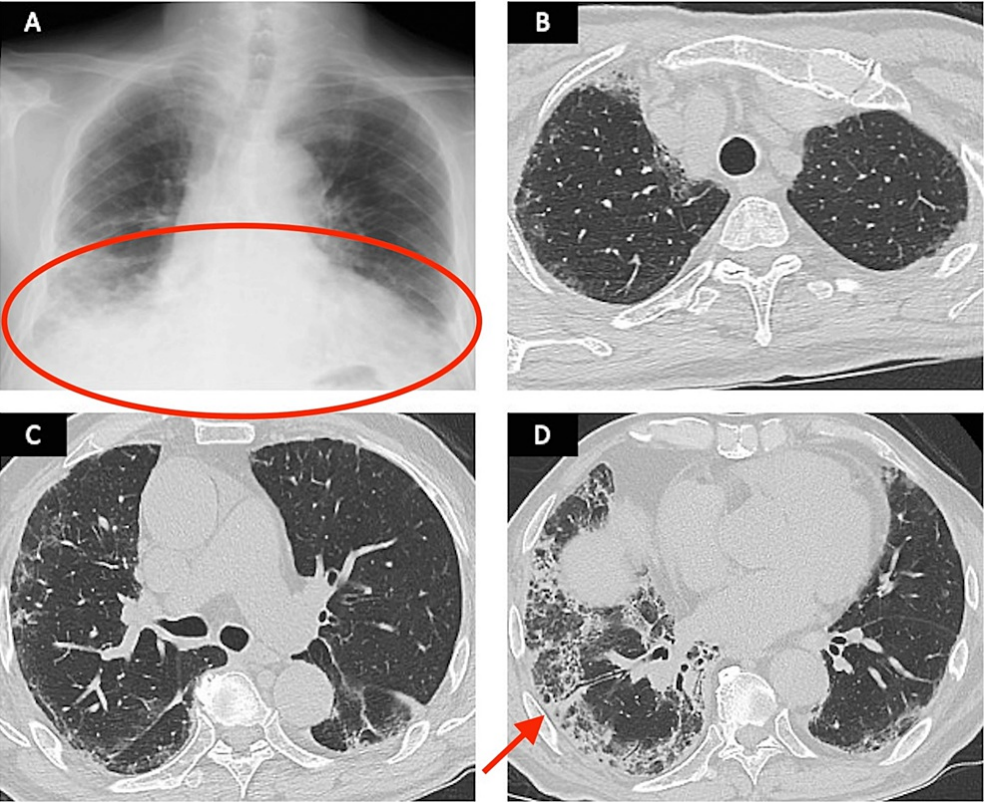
Initial diagnosis imaging examination (A) Chest radiograph showing patchy asymmetrical opacities in bilateral lower zones with septal thickening. (B–D) Chest CT showing bilateral ground-glass opacifications and mild traction bronchiectasis. CT, computed tomography
